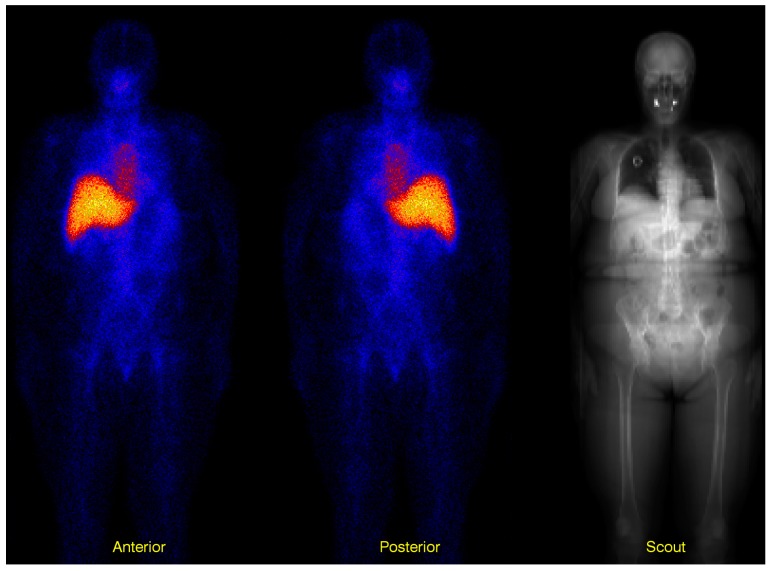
Three images showing anterior and posterior whole-body images. Before applying the geometric-mean on a pixel-by-pixel level, the posterior image is mirrored. The image to the right is the scout image whose intensity describes the amount of photon attenuation in the patient. Bright areas indicate a large probability for photon attenuation. This image is acquired with the X-ray unit, and the values are scaled to represent the attenuation factor for the relevant photon energy. The spatial misregistration between the scout image and the two planar images is accounted for by an image registration procedure, tailored for whole-body images, as described further down in the text.
